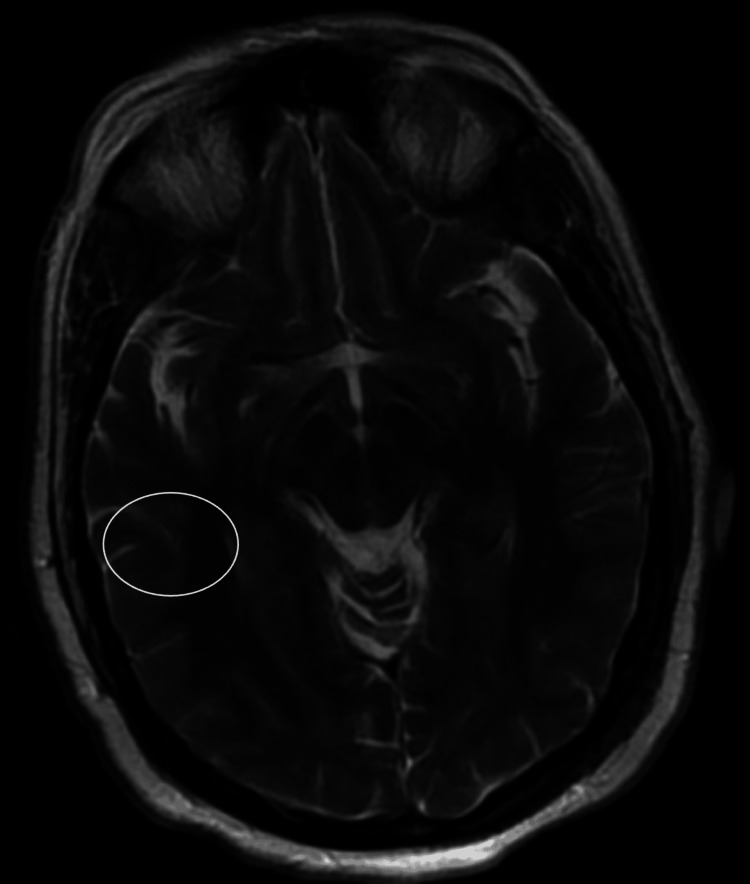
MRI of the brain w/ and w/o contrast T2-weighted MRI of the brain image highlights leptomeningeal enhancement (circled), a sign of meningeal inflammation.
